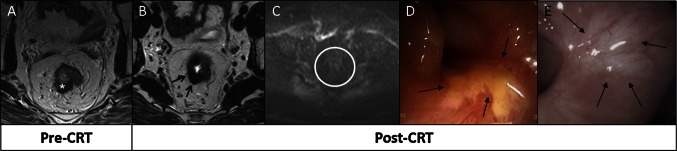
a Tumor (*asterisks*) before CRT. After CRT at T2W-MRI (b), fibrosis (*arrows*) is found with absence of high signal on DWI (c), suggestive of a CR. At endoscopy (d), a residual ulcer (*arrows*) is found, indicating residual tumor. Patient refused surgery and has been followed up for 3.5 years with stable MR image and a healed ulcer (e, *arrows*), so is classified as having experienced CR
